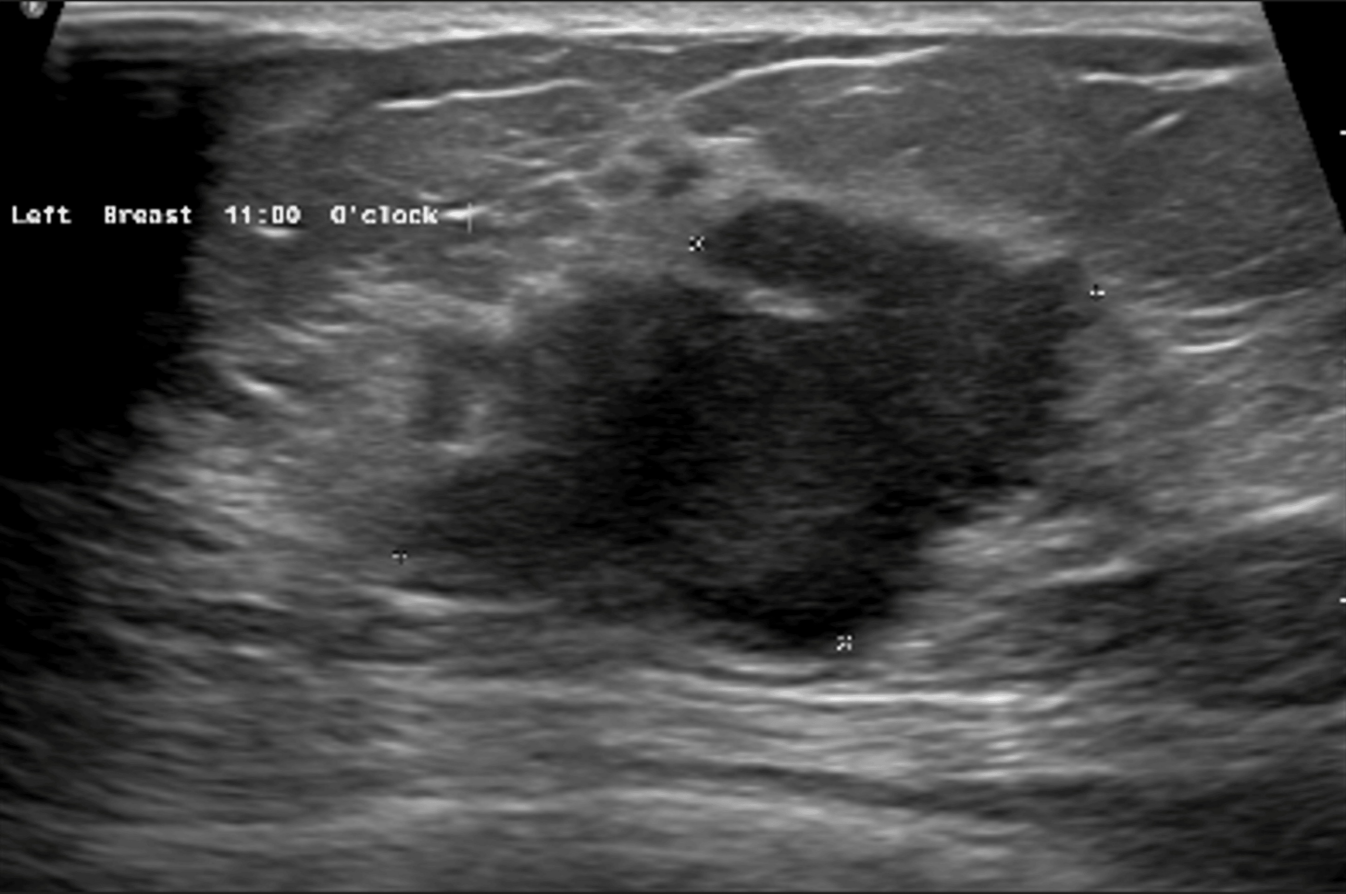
Ultrasonography of the left breast showing a BIRADS 5 lesion in the upper inner quadrant of the left breast BIRADS: Breast Imaging-Reporting and Data System
